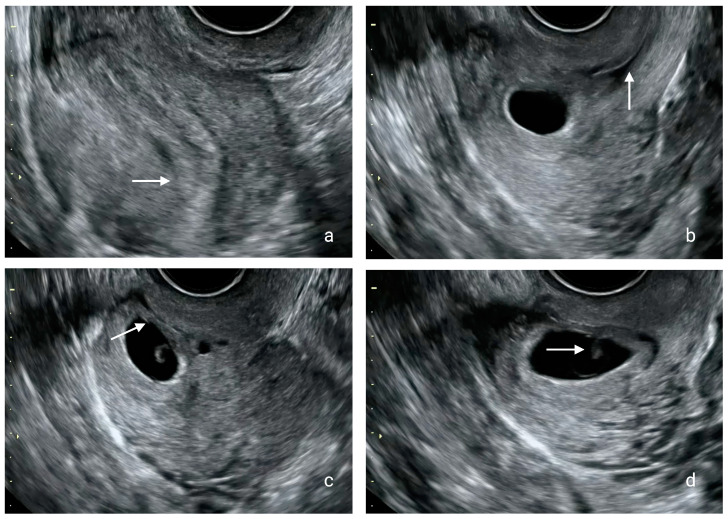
Transvaginal ultrasound findings. (a) The empty uterine cavity marked with a white arrow; (b) the empty cervical canal marked with a white arrow; (c) the gestational sac embedded at the site of the previous lower uterine segment and the thin layer of myometrium between the gestational sac and the bladder marked with a white arrow; (d) the presence of the fetal pole.
